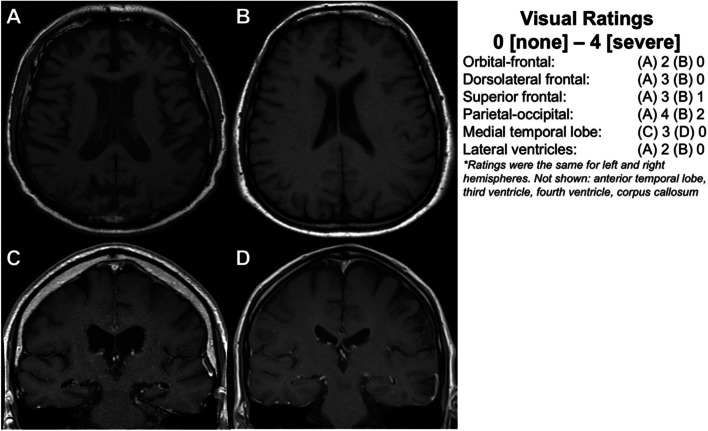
Antemortem MRI scans for brain donors with autopsy-confirmed CTE compared to participants with normal cognition. Three neuroradiologists used established visual rating scales to rate patterns of frontal, anterior temporal, parietal-occipital lobe atrophy on axial T1 sequences, as well as medial temporal lobe atrophy on coronal sequences in brain donors with CTE and participants with normal cognition. The regions were rated on a 5-point scale with 0 = none and 4 = severe. A Axial T1 of a male former professional American football player in his early 60’s with CTE stage IV that was rated to have mild orbital-frontal and anterior temporal lobe atrophy (not shown), moderate dorsolateral and superior frontal lobe atrophy, severe parietal-occipital lobe atrophy, and presence of an anterior and posterior cavum septum pellucidum. B Axial T1 of a participant with normal cognition in his late 60’s rated to have no orbital-frontal, dorsolateral frontal, or anterior temporal lobe (not shown) cortical atrophy; minimal superior frontal atrophy; mild parietal-occipital lobe atrophy; and absence of a cavum septum pellucidum. C and D are coronal sequences that show moderate hippocampal atrophy in a former professional American football player in his early 80’s with CTE stage IV (C) compared to no hippocampal atrophy in a participant with normal cognition in his early to mid-70s (D)
